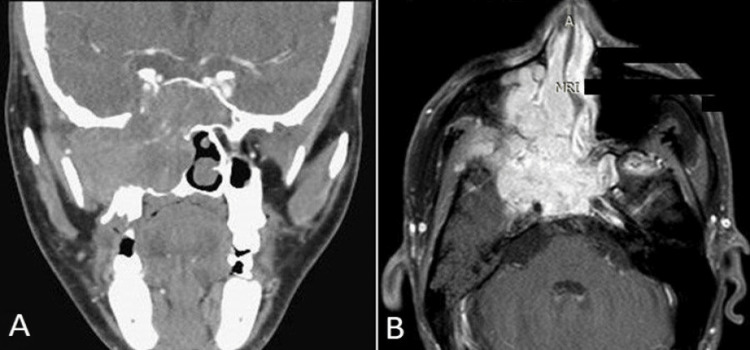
CT and MRI findings. (a) Coronal CT image showing a mass occupying the right nasal cavity with extensions into the parapharyngeal space, infratemporal fossa, and skull base. (b) Axial MRI image depicting the tumor encasing the right internal carotid artery.
